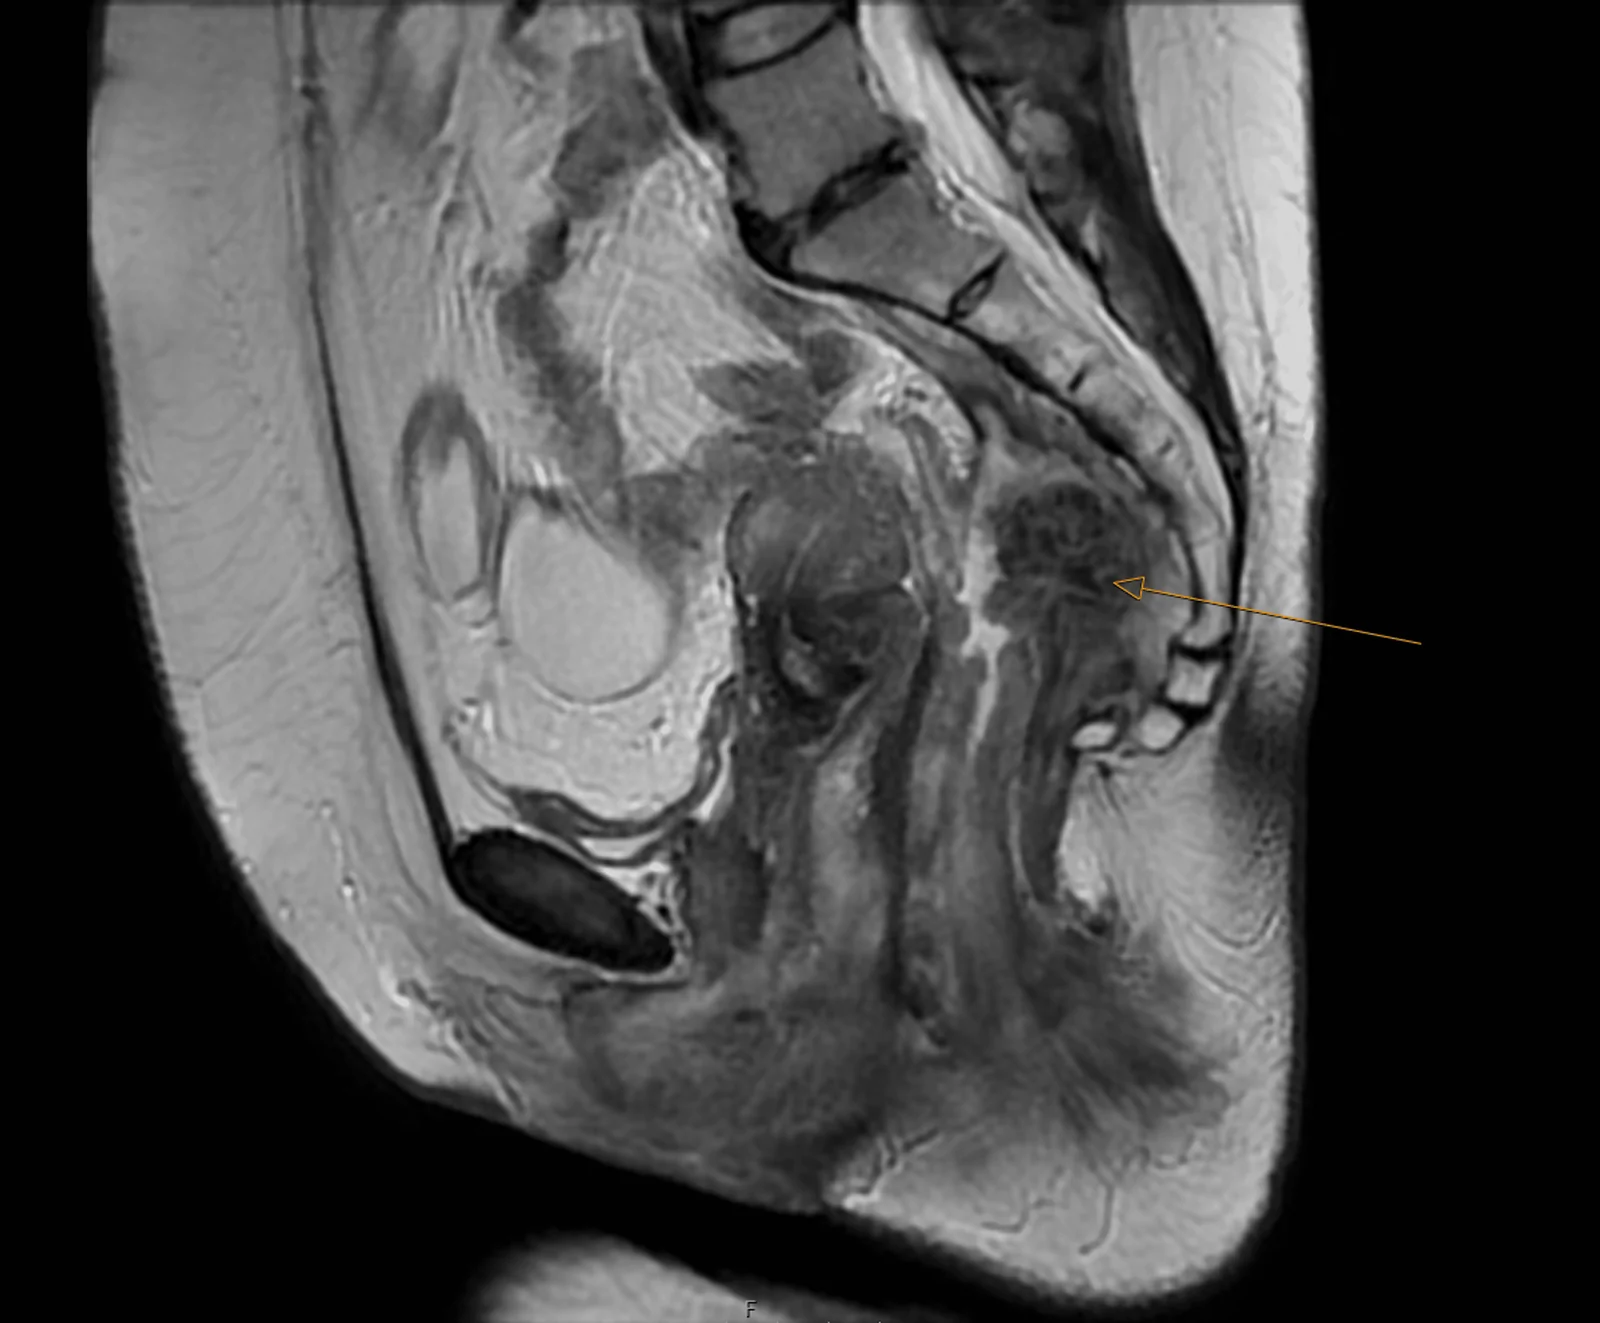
Sagittal pelvic MRI demonstrating a presacral collection at the colorectal anastomotic site (arrow)
